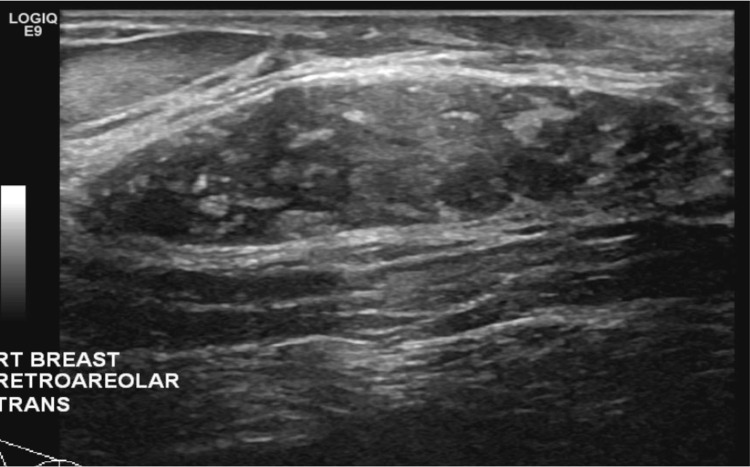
Ultrasound of the right breast (transverse view). Targeted ultrasound demonstrates an isoechoic, oval mass with circumscribed margins measuring 51 × 13 × 47 mm at the site of the mammographic mass in the subareolar region. Ultrasound of the axilla demonstrates morphologically normal lymph nodes.
